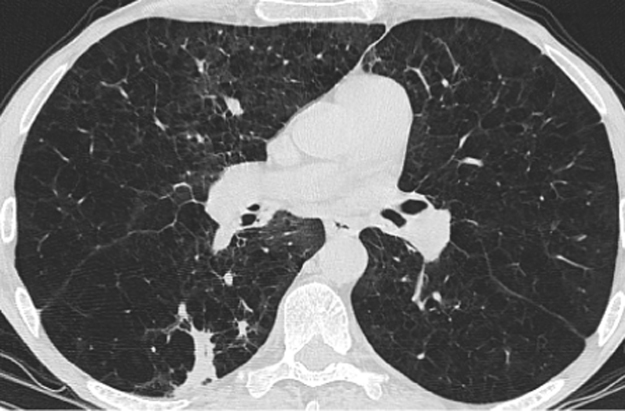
CT thorax from November 2016 showing new subpleural consolidation in right lobe.
